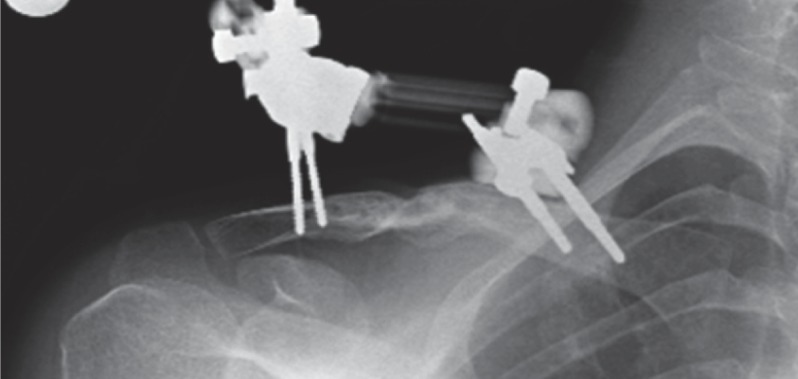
Radiograph of the clavicle 3.5 months after application of the external fixator, showing callus formation at the fracture site.
